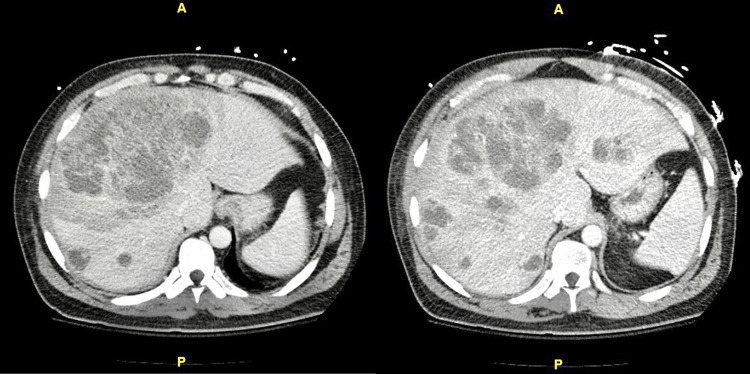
Computed tomography of the abdomen showing innumerable low-density hepatic lesions throughout with the largest complex lesion centered about the right lobe measuring 12 cm x 16 cm.
